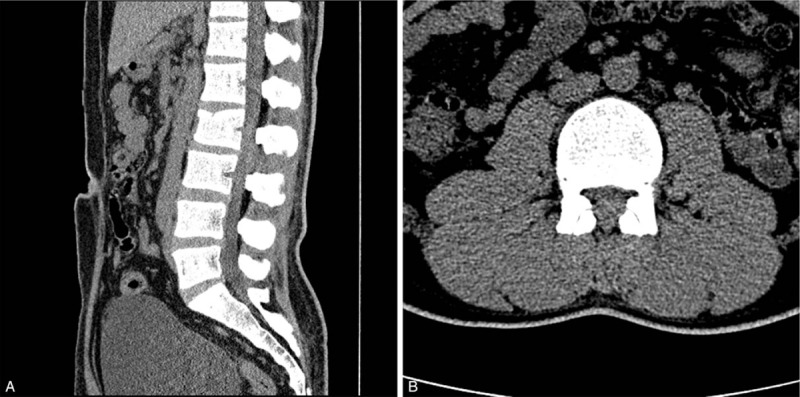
Initial lumbar spine computed tomography scan (A and B) showing a focal compression fracture of the L2 body at the upper anterior portion and a suspected hematoma in the posterior epidural region at L2-L3.
